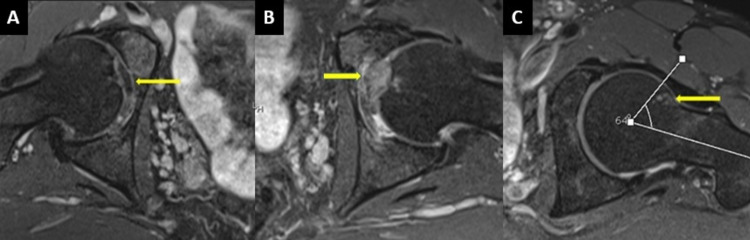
MRI (oblique axial PD fat saturation) image of a 47-year-old male who presented with chronic left-sided hip pain. (A) Normal ligamentum teres on the right side (yellow arrow). (B) Left ligamentum teres edema and hypertrophy. (C) The alpha angle is increased by 64 degrees, and fibrocystic changes are seen in the head-neck junction with a small bony bump in the left hip. MRI: magnetic resonance imaging; PD: proton density
